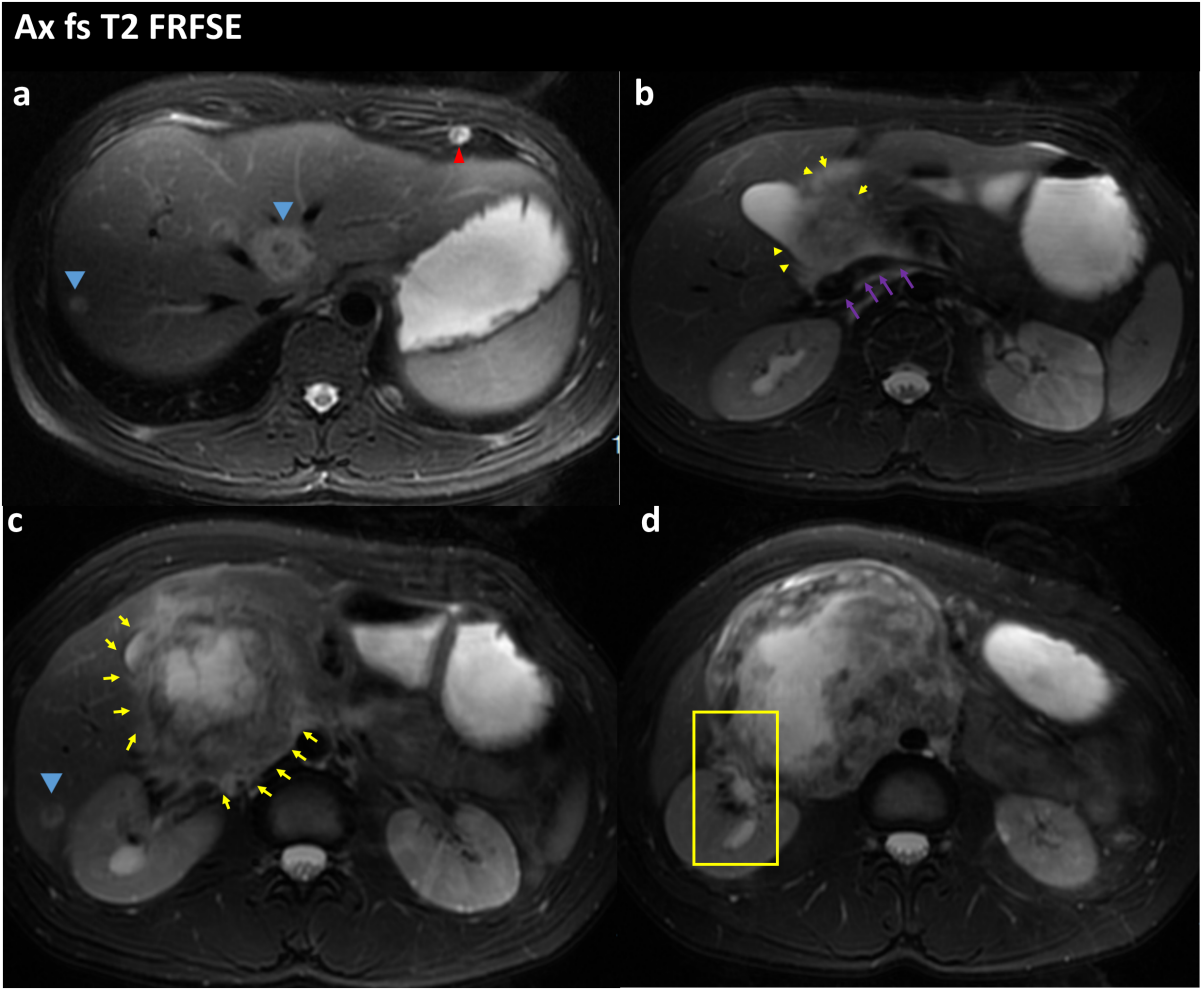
FS-T2WI image of the patient’s abdominal MRI on admission; the yellow arrow points to the location of the abdominal tumor; the blue arrow points to the liver metastases; the red arrow points to the lung metastases; the purple arrow points to the location of the inferior vena cava; (A) Tumor with multiple liver and lung metastases. (B) the tumor is seen encircling the inferior vena cava and is poorly demarcated from the inferior vena cava. (C) Tumor is in the form of an irregular lump, with internal heterogeneous enhancement and blurred margins. (D) the right renal pelvis is seen in the yellow box and is tortuously dilated and poorly demarcated from the tumor.
